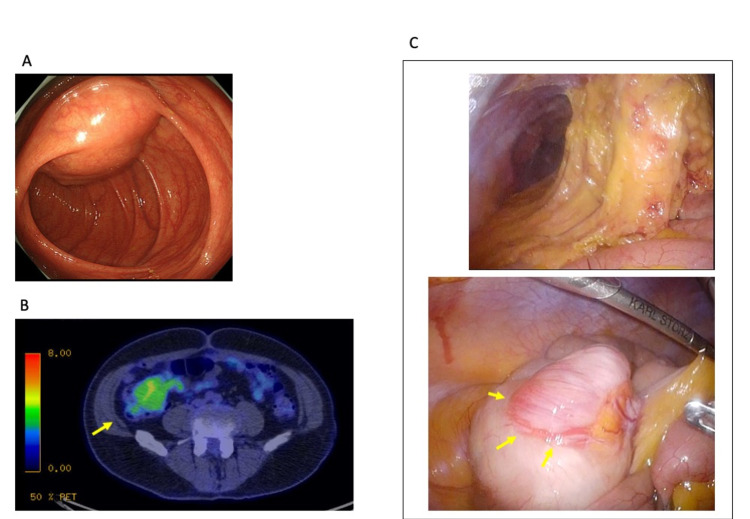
Colonoscopy/18F-FDG positron-emission tomography-CT/laparoscopic findings A: Colonoscopy. Total colonoscopy found a submucosal tumor with cushion sign in the cecam. B: 18F-FDG Positron-emission tomography (PET) CT. 18F-FDG PET-CT showed a mild accumulation of the 18F-FDG in the tumor site (SUVmax = 5.0) (arrow). Distant metastasis was not evident in the study. C: Intraoperative pictures (laparoscopic pictures). Laparoscopically, intraabdominal adhesion was seen mostly upper part of the abdominal cavity due to prior surgery (laparoscopic distal gastrectomy). In contrast, intraabdominal adhesion was not seen around the ileocecal parts. The tumor protruding from the cecal wall was seen (arrows).
